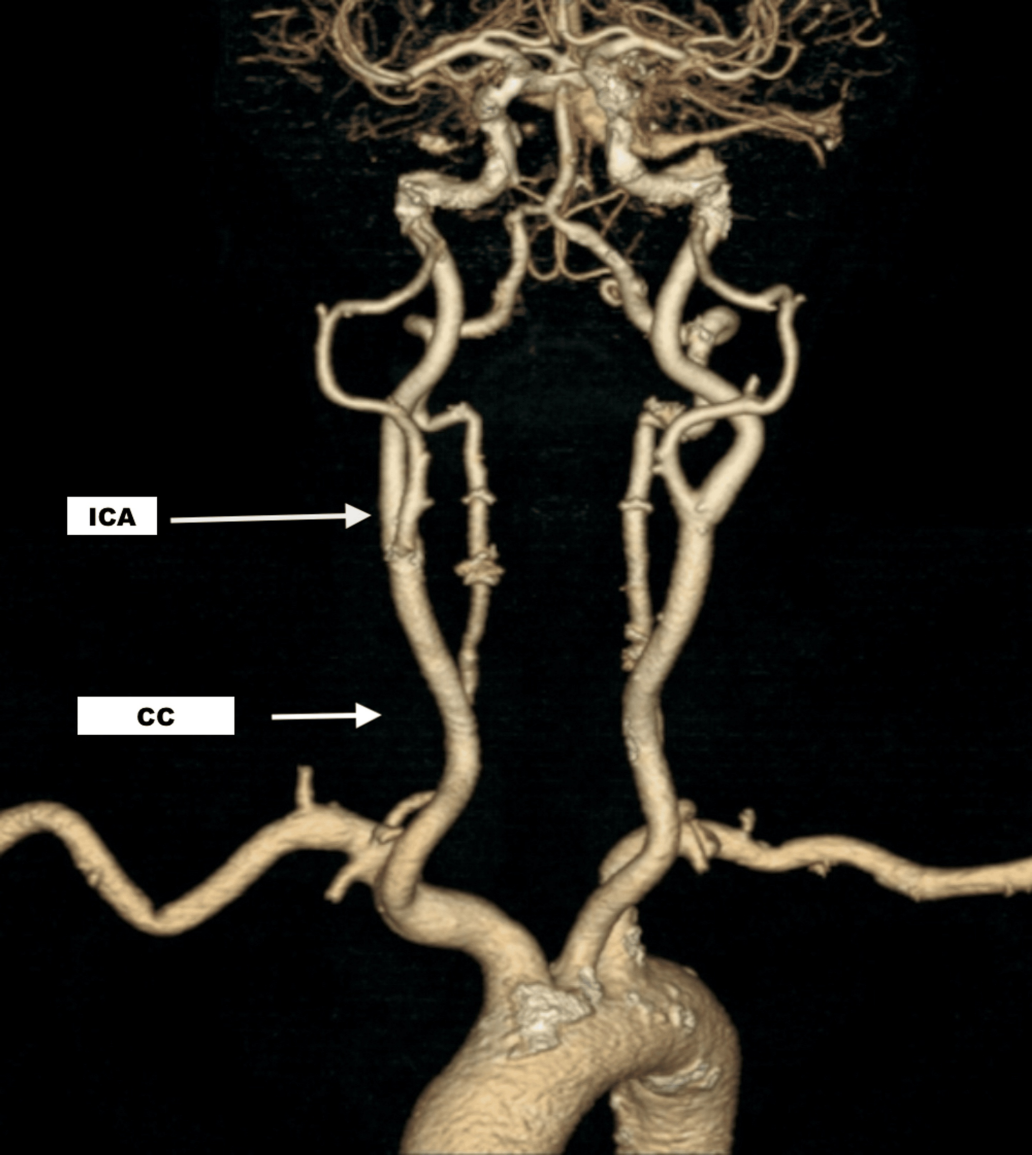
CTA neck (axial) showing the absence of significant occlusion in the common and internal carotid arteries CTA: computed tomography angiogram; ICA: internal carotid artery; CC: common carotid artery
